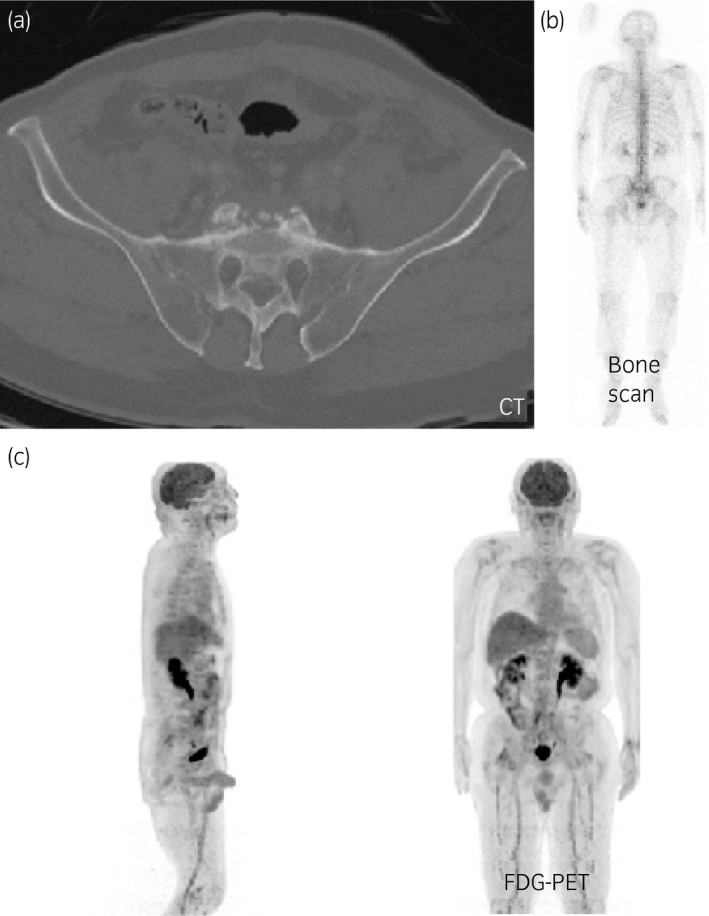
(a) CT, (b) bone scintigraphy, and (c) FDG‐PET findings when the re‐elevation of HCG levels was observed.
